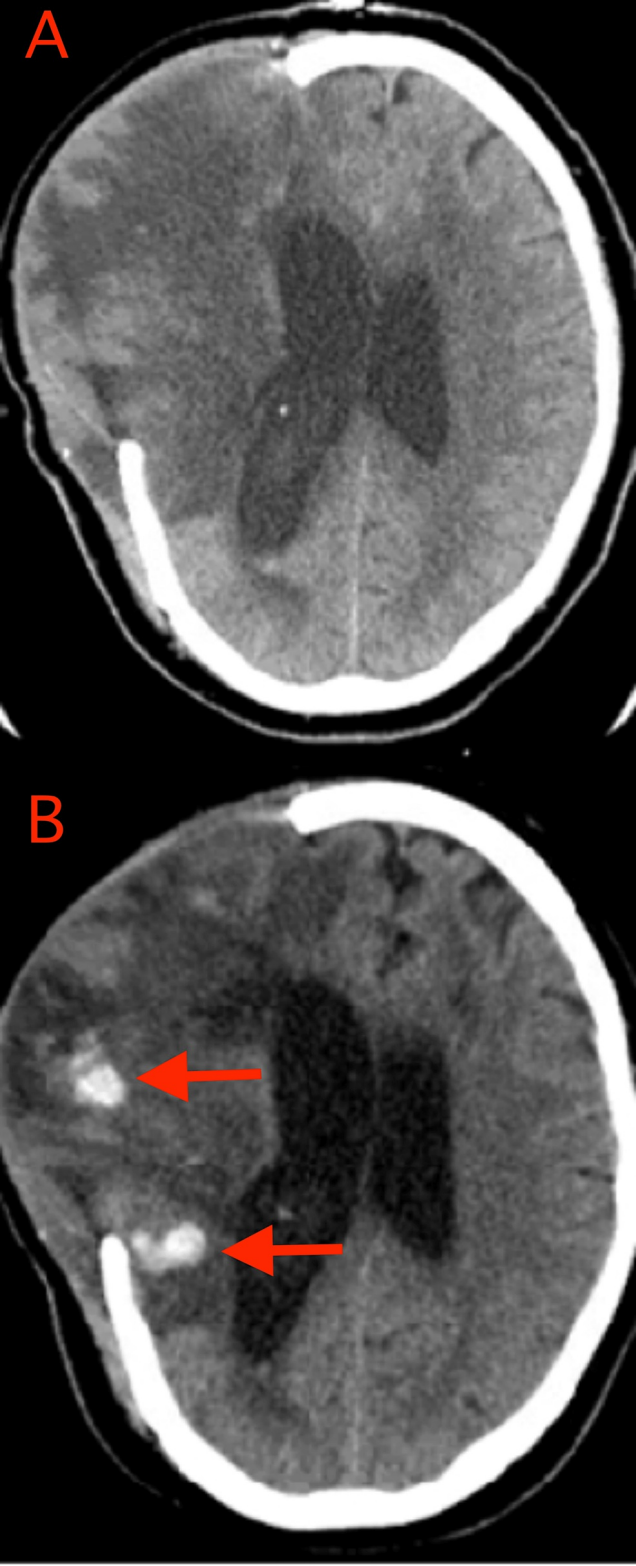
CT images demonstrating DTICH type 1B CT images on POD 22 (top panel A) and 25 (bottom panel B) for a patient experiencing DTICH type 1B are shown. The bottom image shows small hematomas within the frontal and temporal lobes (red arrows in panel B) not seen on imaging three days prior. CT: computerized tomography; POD: post-operative day; DTICH: delayed traumatic intracerebral hematoma
